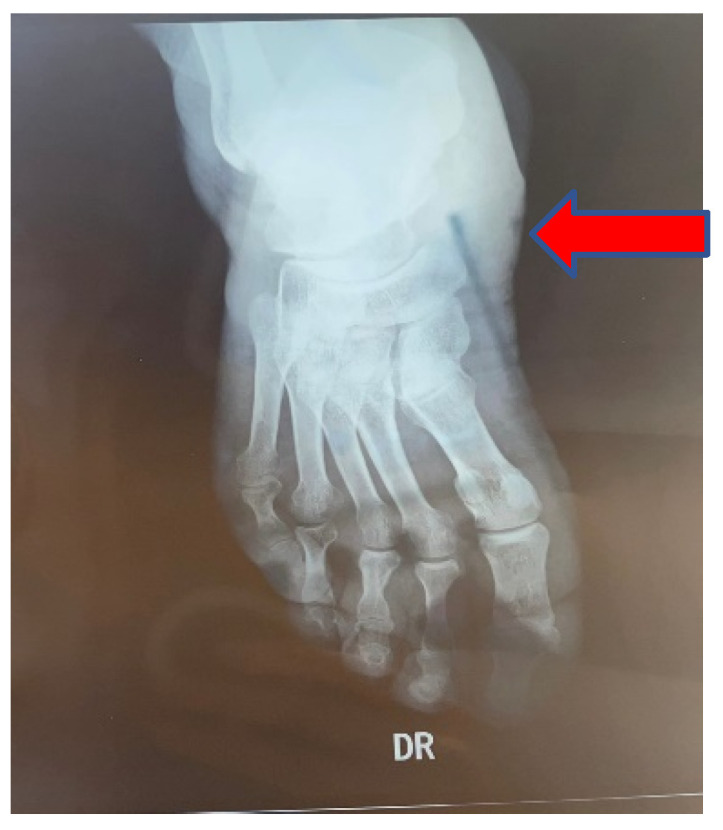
Radiograph of the right foot in a diabetic patient involving zone II according to the Sanders–Frykberg Classification, Dr. Ion Cantacuzino Hospital Photo Archive.
